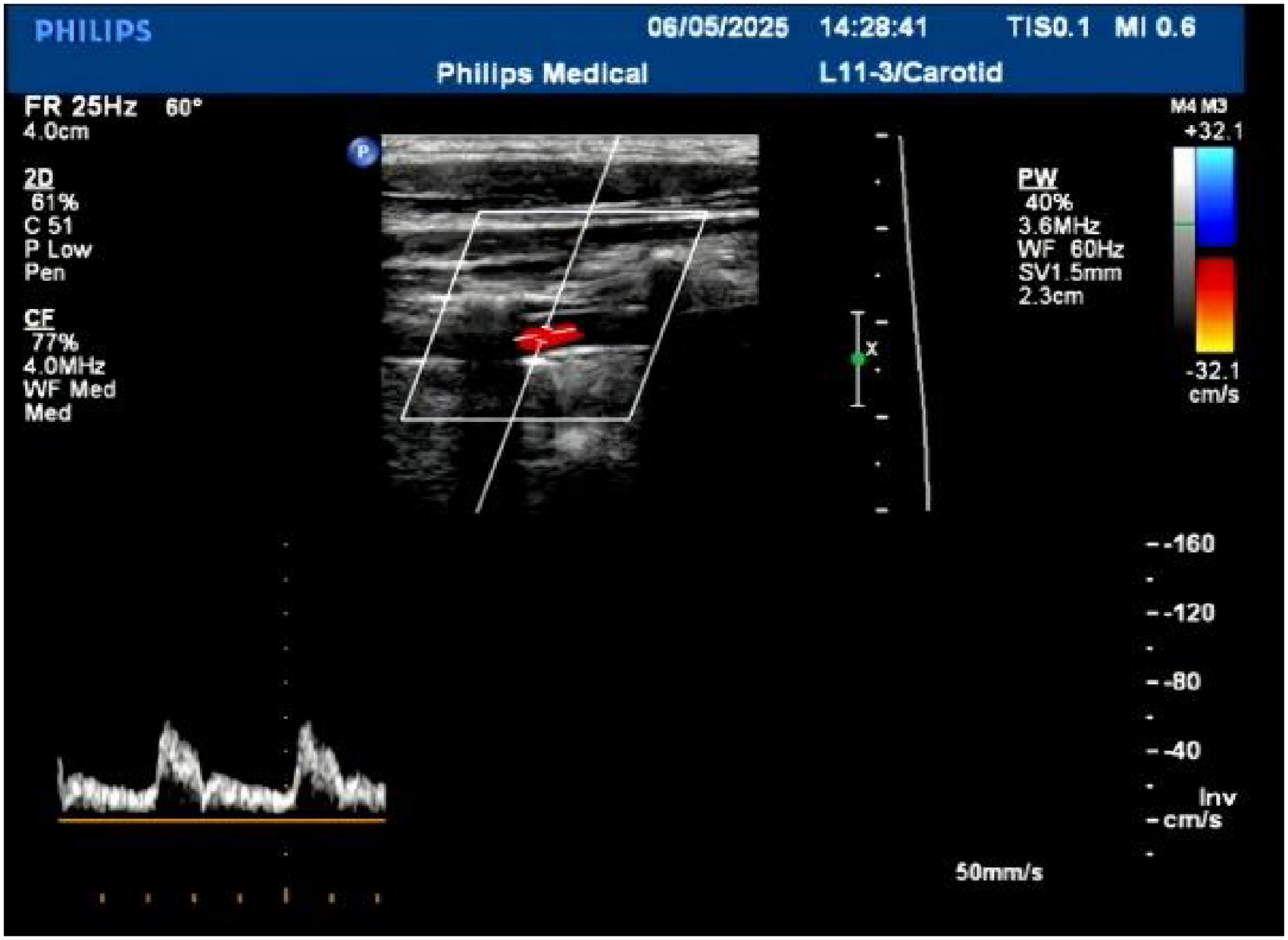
Neck vascular ultrasound.
